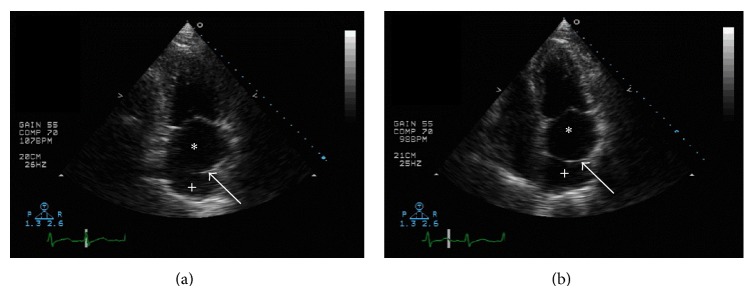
Transthoracic echocardiogram: 2-chamber view (a) and 4-chamber view (b) showing the left atrium divided into proximal (+) and distal (∗) chambers by a fenestrated membrane (arrow).
